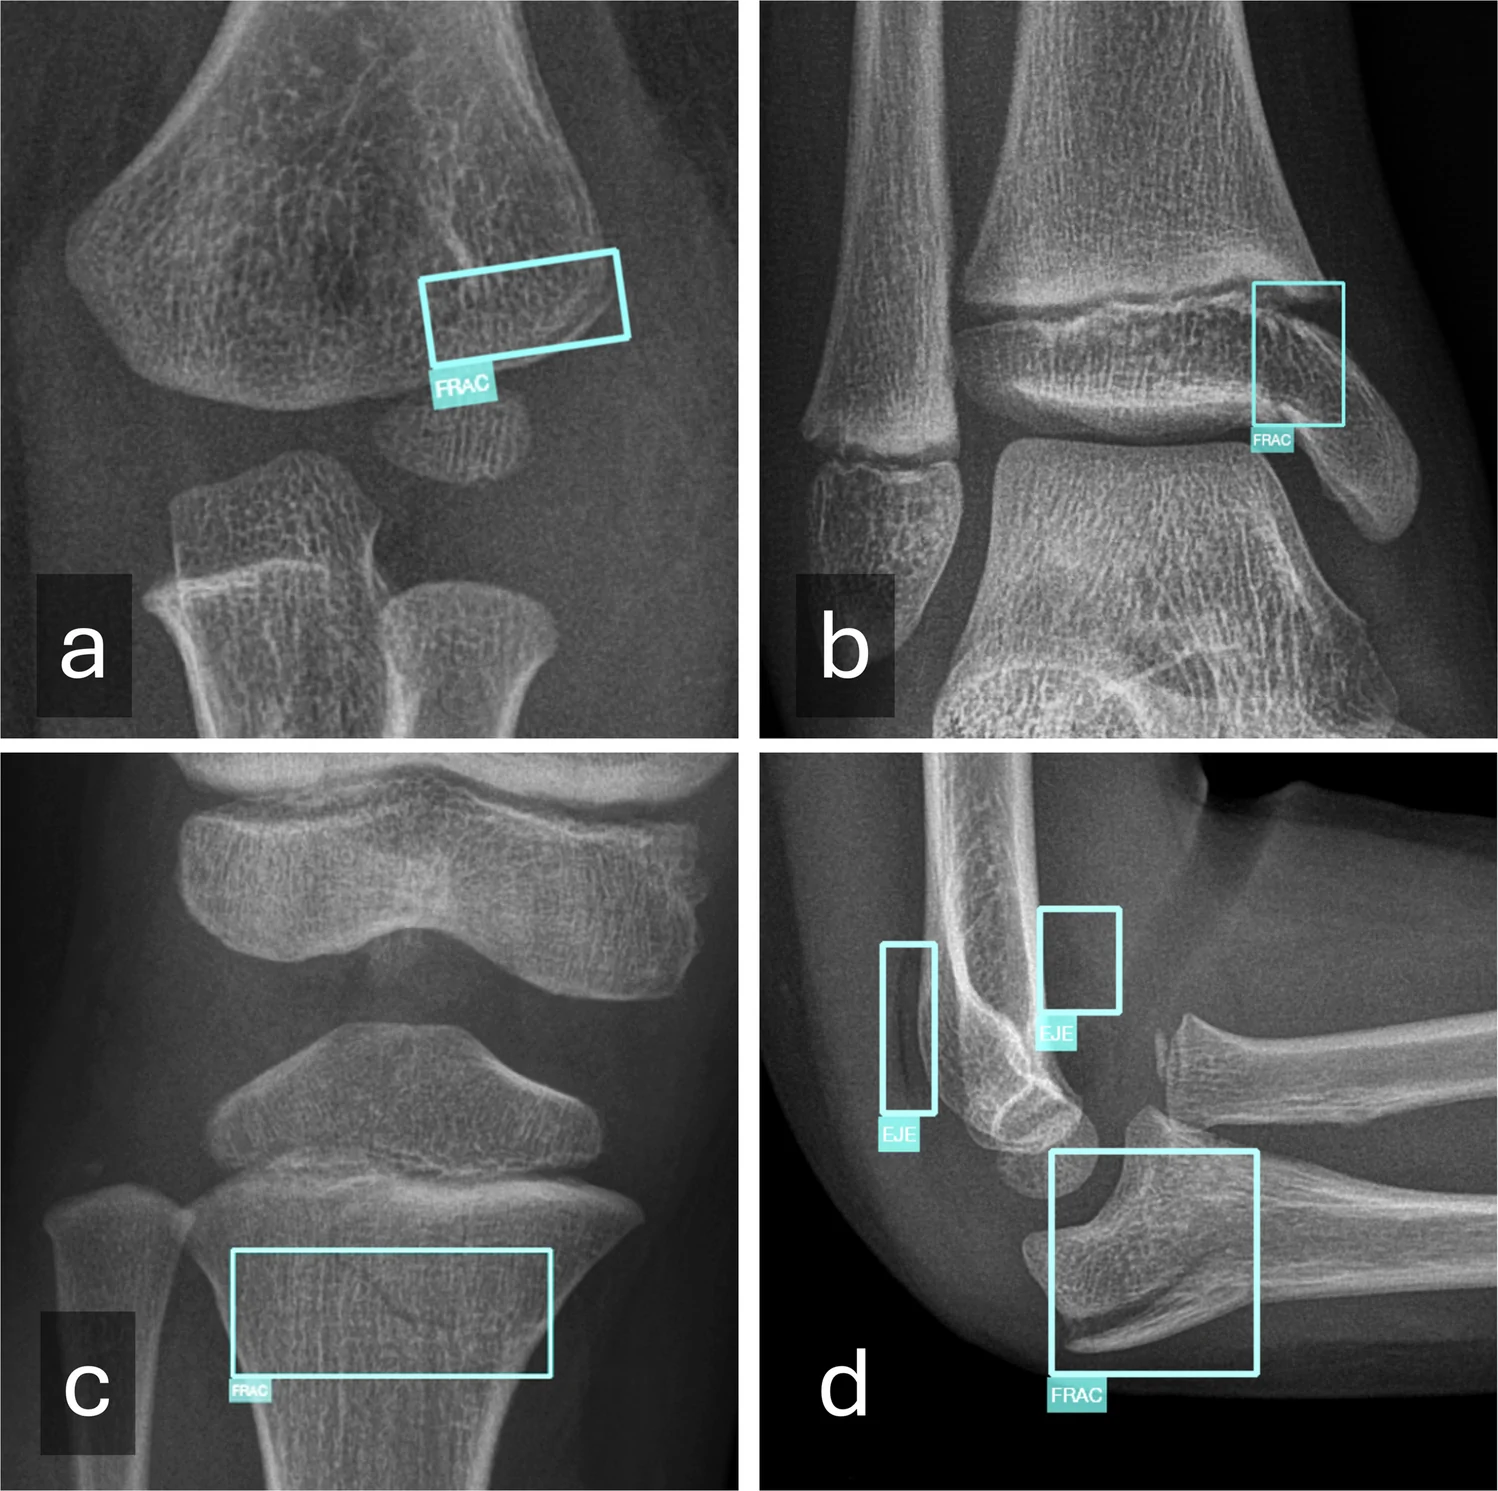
Representative radiographs of four medicolegally relevant paediatric fractures (FRAC): (a) lateral condyle fracture of the humerus (3-year-old girl, posteroanterior view), (b) medial malleolus fracture (9-year-old boy, posteroanterior view), (c) trampoline fractures of the proximal tibia (4-year-old boy, posteroanterior view), and (d) Monteggia fracture with elbow joint effusion (EJE) (5-year-old girl, lateral view). Fractures in (a–c) are correctly detected and highlighted by the artificial intelligence using bounding boxes. In (d), the system identifies the ulnar fracture and joint effusion but fails to detect the radial head dislocation
